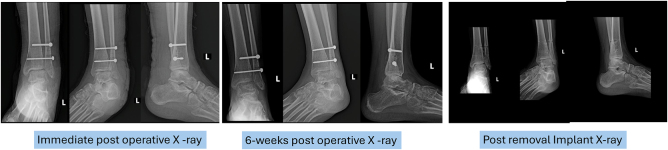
Postoperative X-ray: The immediate postoperative X-ray, the X-ray taken 6 weeks postoperatively, and the X-ray taken after implant removal all show satisfactory anatomical alignment..
